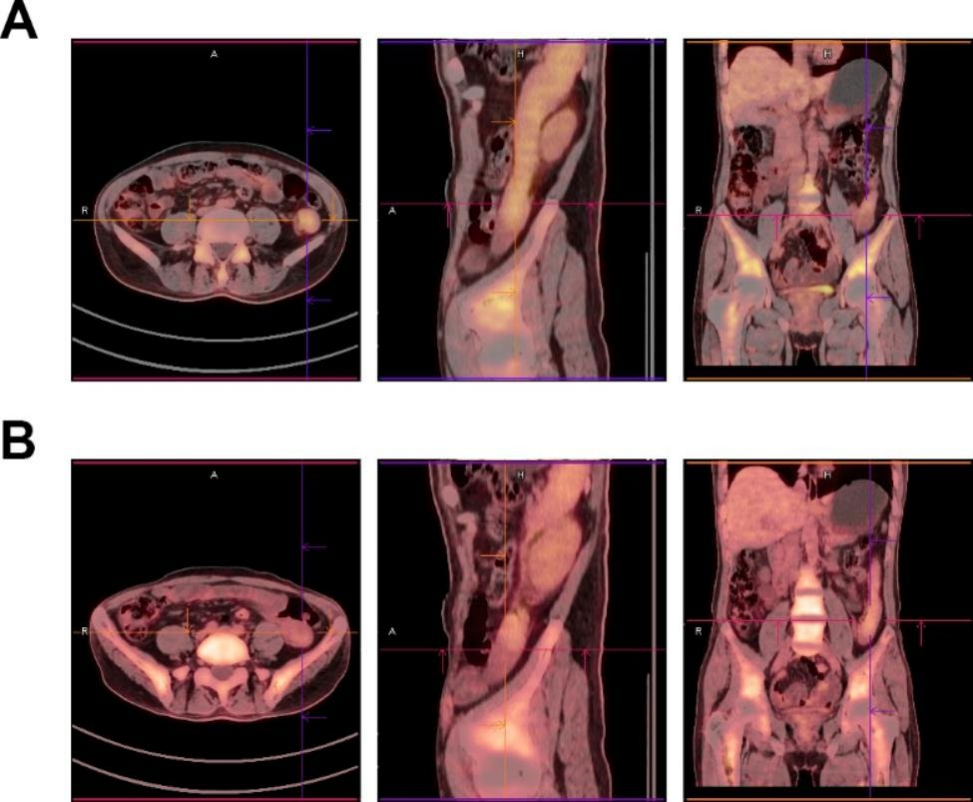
PET/CT after chemotherapy showed that the volume of both intra-abdominal tumor and retroperitoneal lymph nodes is smaller than before. (A) after two cycles; (B) after four cycles
